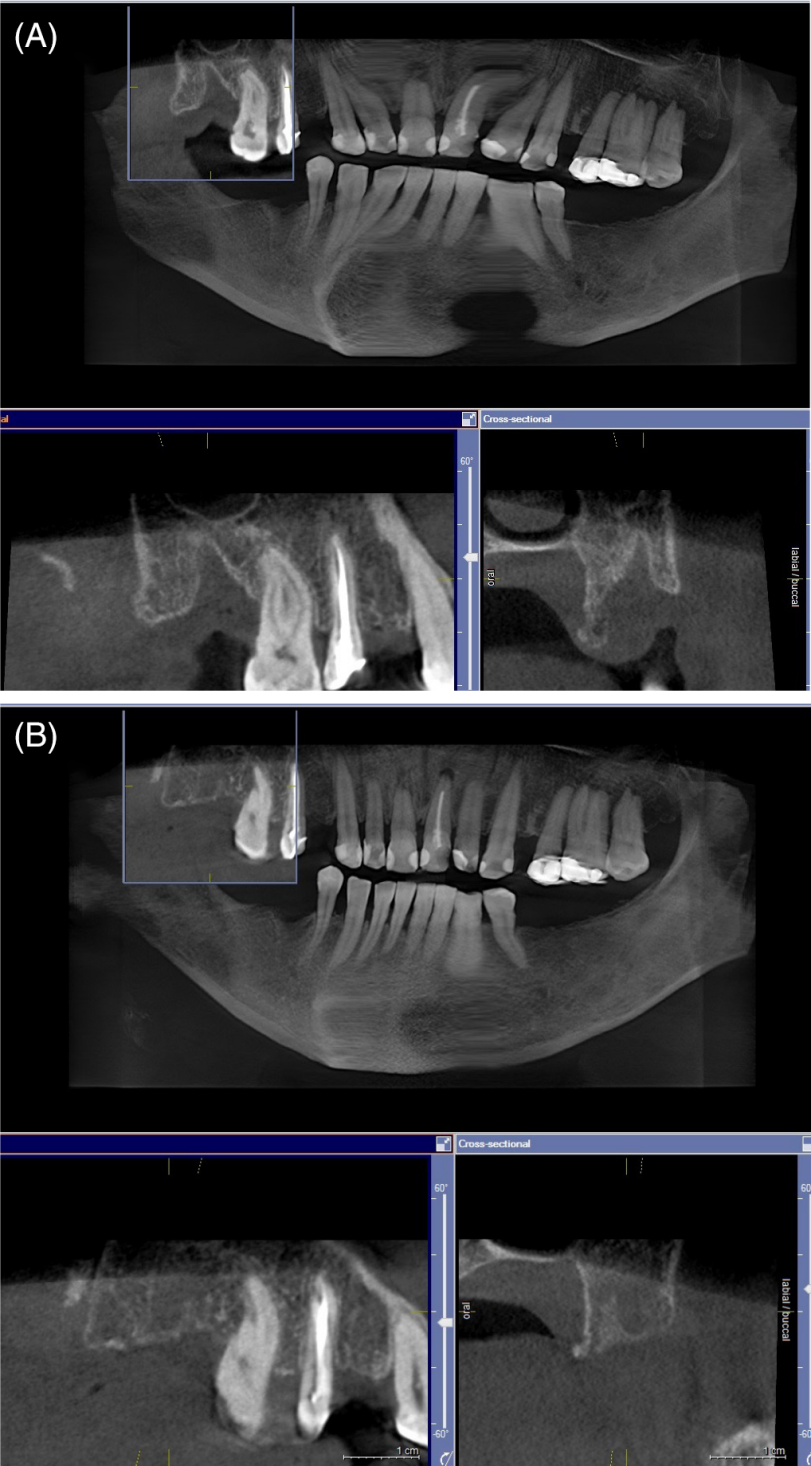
Same patient as Figure 4, extraction of 18 due to chronic periodontitis. Standard extraction procedure was used. CBCT scan 1 day after extraction (A) and at for month follow up (B)
